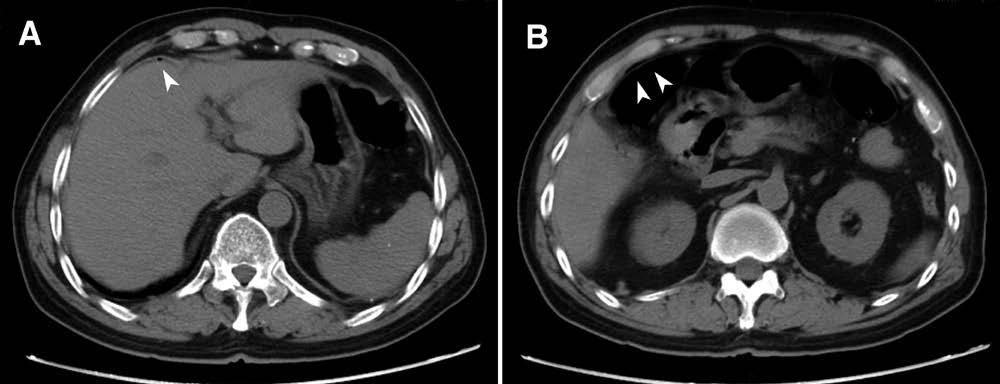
Urgent abdominal computed tomography scans showed a few bubbles on the frontal surface around the liver (A, arrowhead) and a little extraluminal free air in the upper abdomen (B, arrowhead). No peritoneal effusion was found.
